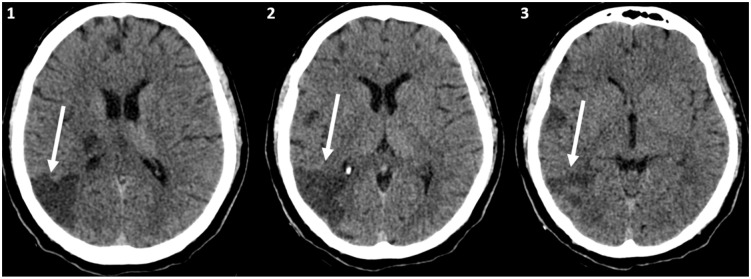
CT angiography of the right upper limb showed a filling defect in the distal brachial artery
